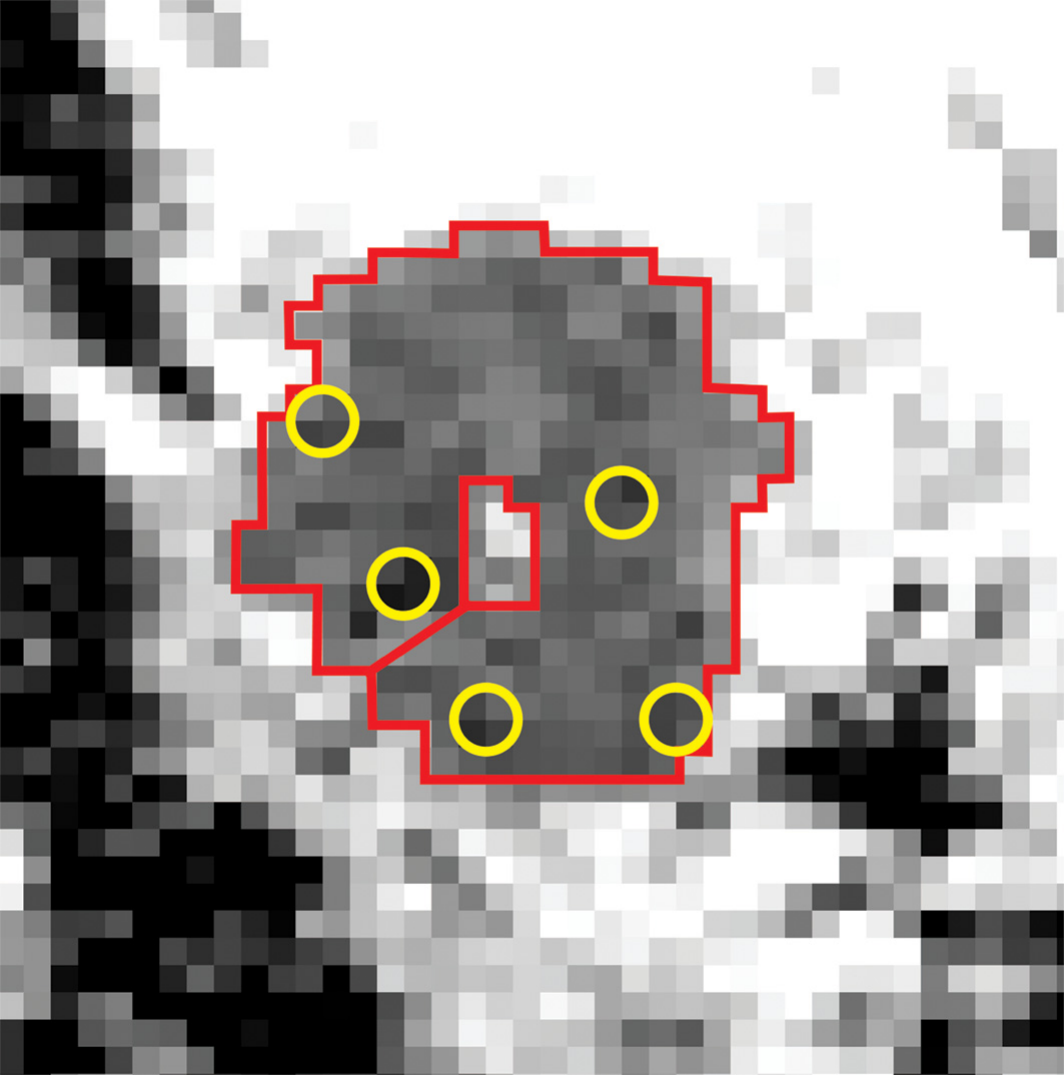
Whole lesion (*red*) and small (*yellow*) region of interest (ROI) placement on a diffusion-weighted image (DWI) of the right breast of a 41-year-old woman.
